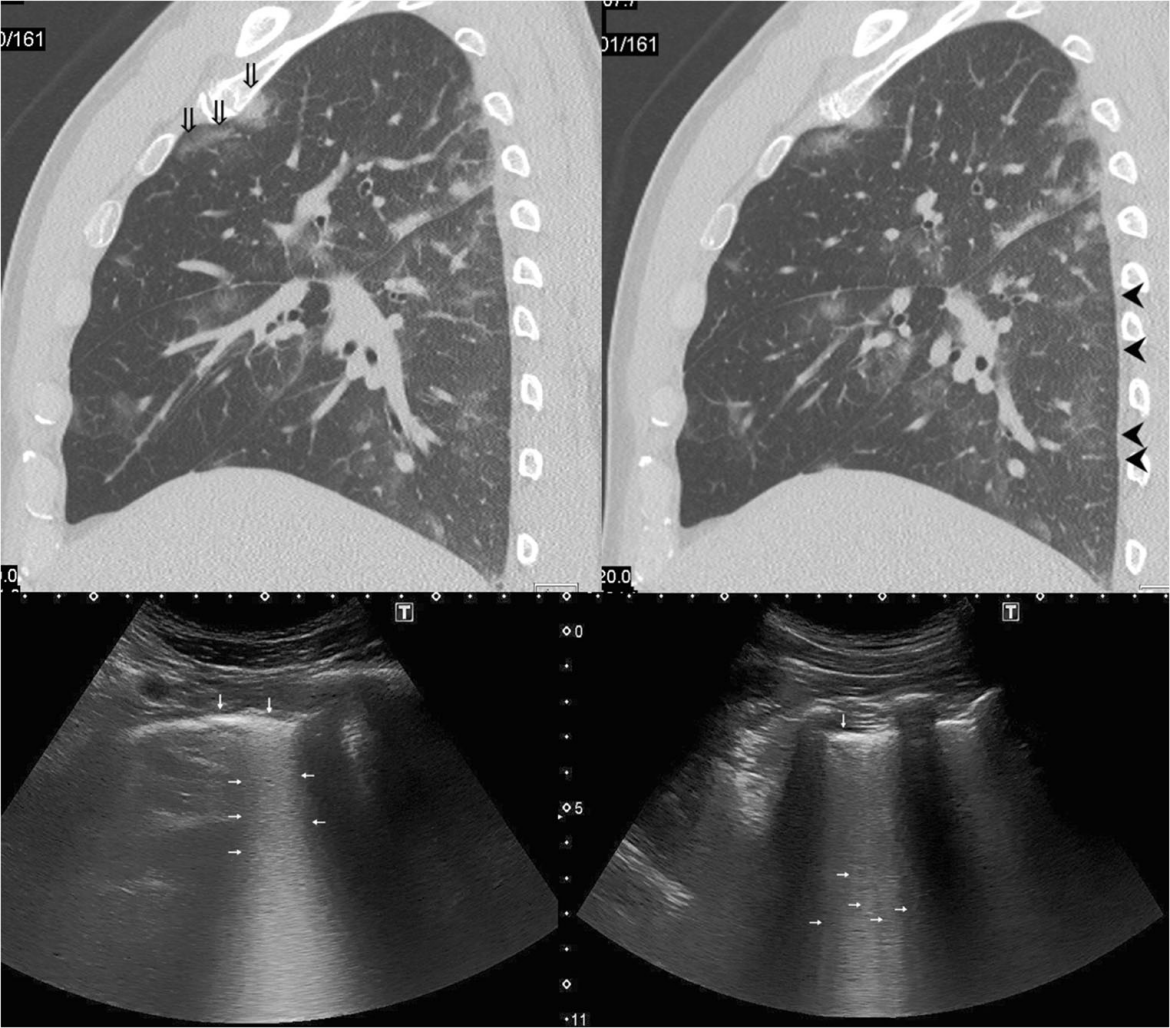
Comparison of exemplary lesions on CT and LUS. Left: GGO in the upper lobe of the right lung (double arrows) yield very densely converging B-Lines (horizontal arrows) that seem to merge into one broad, echogenic vertical artifact arising from the irregular pleural line (vertical arrows). Right: Thickened interlobular septa (arrowheads) are visible on CT; they correlate to B-Lines in LUS (horizontal arrows) that are still distinguishable from one another. The density of B-Lines seems to correlate to the extent of thickening in interlobular septa [7]. All images were obtained on day 17 after symptom onset
